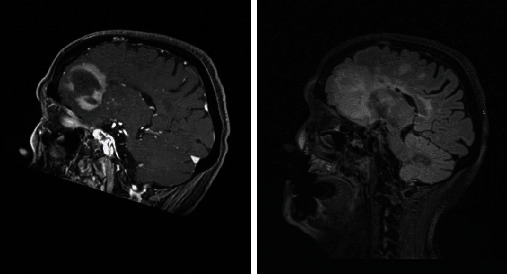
Extensive white matter changes with multiple patchy areas of intense enhancement in the left anterior and inferior frontal lobes. Radiographic differential diagnosis: PCNSL, high-grade primary neoplasm, leukoencephalopathy, and primary leukoencephalopathy with immune-reconstitution inflammatory syndrome.
